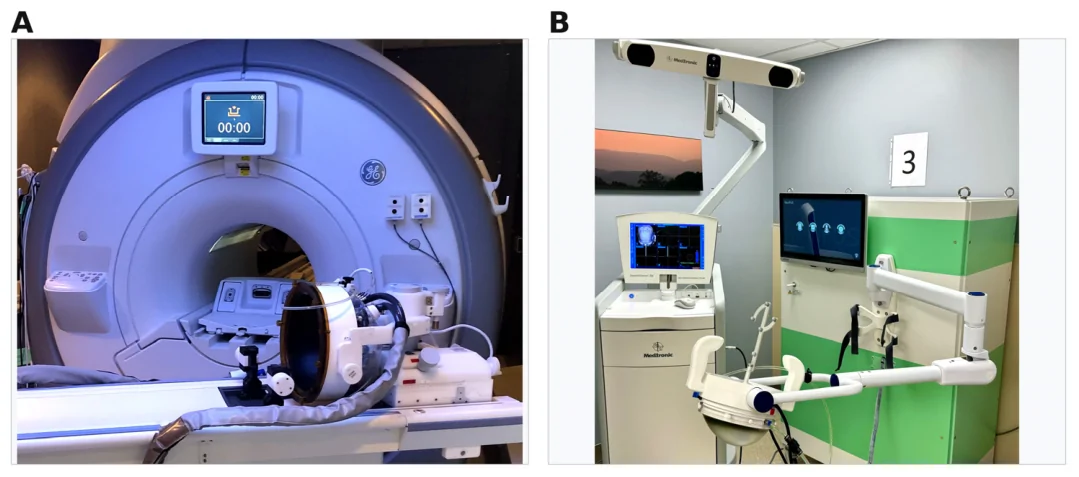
Focused ultrasound technology. Representative focused ultrasound (FUS) technologies used in active brain-cancer clinical trials. (A) The Insightec ExAblate transcranial MR-guided FUS system. A hemispheric phased-array helmet (foreground) is coupled to the scalp via degassed circulating water and operated within an MRI bore, enabling real-time anatomic targeting, MR thermometry and post-treatment confirmation of blood–brain barrier opening on contrast-enhanced T1 imaging. (B) The NaviFUS neuronavigation-guided low-intensity FUS system, shown in a procedure room. The platform integrates a 256-element phased-array transducer (foreground) with optical neuronavigation tracking (Medtronic StealthStation, Medtronic Corp, Minneapolis, MN, USA, upper left), co-registered to the patient’s MRI, allowing treatments to be delivered outside an MRI environment. SonoCloud-9 is not depicted here, as it is a fully implanted intracranial device without an external form factor comparable to panels (A,B); see Table 1 for a full comparative summary of all three platforms, including guidance modality, invasiveness, skull attenuation, treatment paradigm, and clinical indications.
